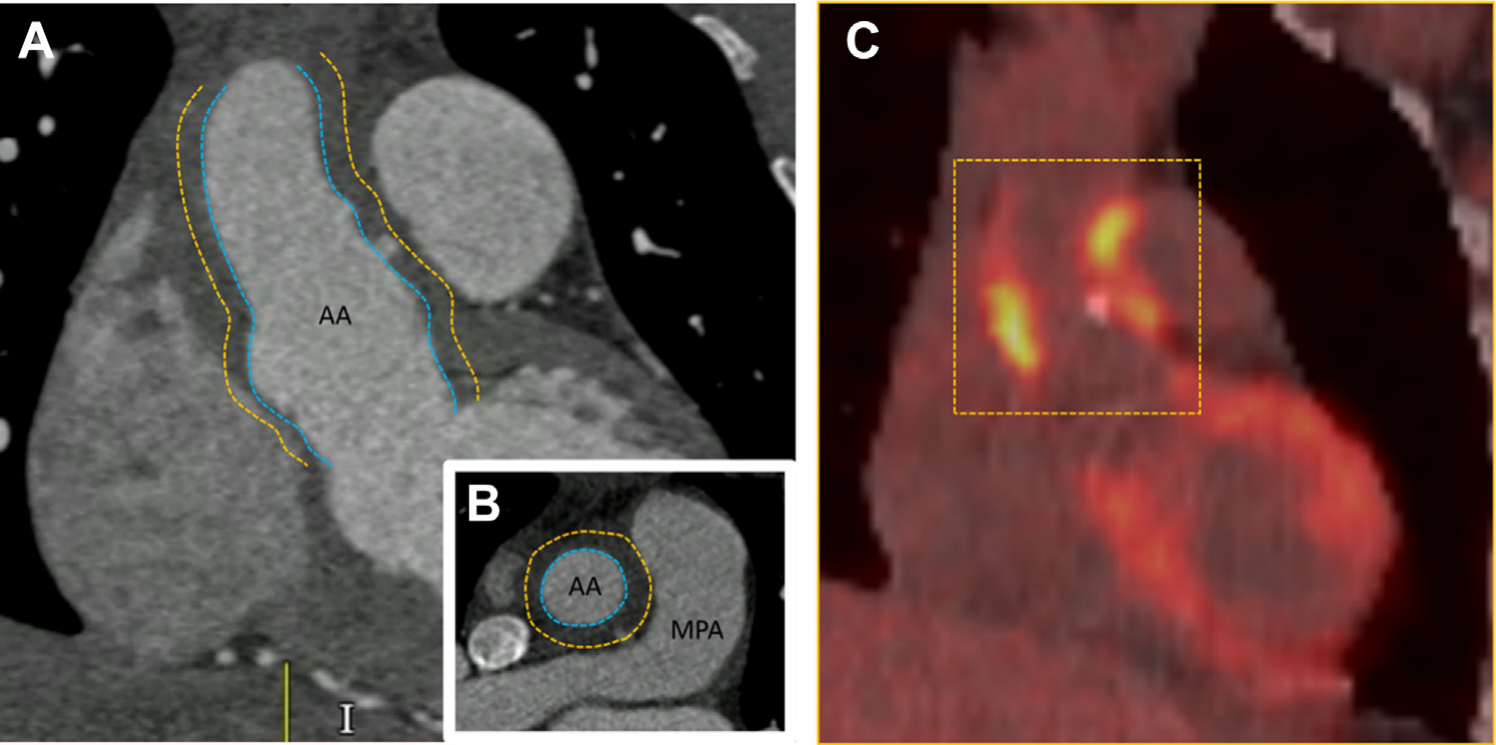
▪▪▪ (A and B) Coronal (A) and axial (B) CT images of extensive soft tissue thickening seen in the aortic root and ascending aorta (true lumen = blue dotted lines; extent of thickening = orange dotted lines). (C) PET-CT images of the corresponding section of ascending aorta with evidence of active irregular soft tissue (orange dotted box) consistent with extensive inflammation. AA = ascending aorta; CT = computed tomography; MPA = main pulmonary artery; PET = positron emission tomography.
